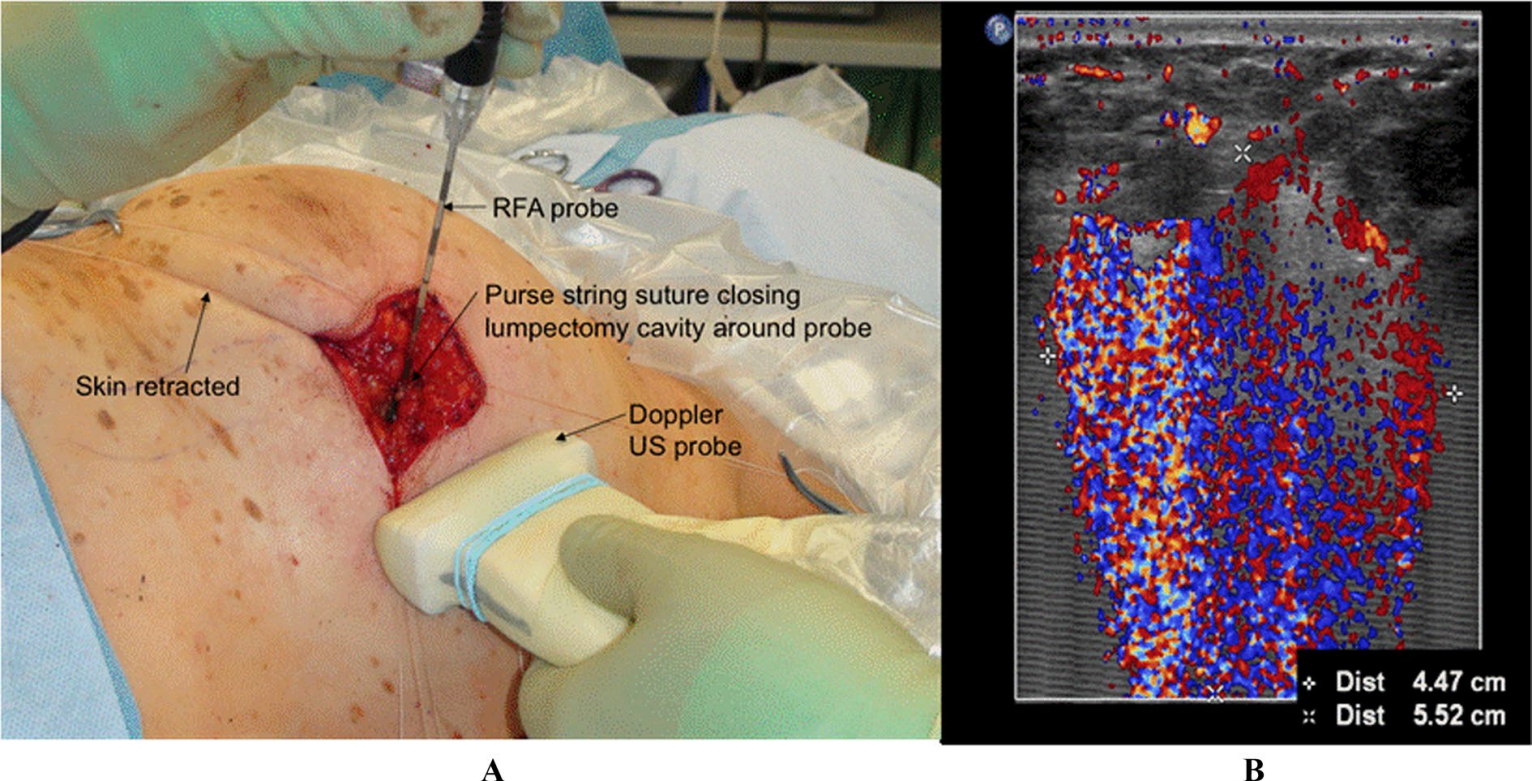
eRFA technique with intraoperative monitoring of the ablation zone; A intraoperative setup with a 12 MHz ultrasound probe used in Doppler mode for measuring ablation zone by visualizing movement of tissue caused by off-gassing of nitrogen; B Doppler signals that demonstrate an ablation zone of 4.47 cm (denoted by +) by 5.52 cm (denoted by *x*)
